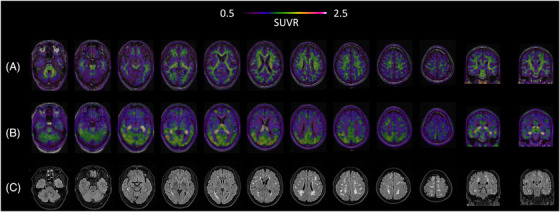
Brain imaging findings in a patient carrying p.K687Q *APP* mutation. (A) ^18^F‐florbetapir amyloid PET showing negative results. (B) ^18^F‐Florzolotau PET demonstrating asymmetric cortical uptake (SUVR maps overlaid on structural MRI). (C) T2‐FLAIR MRI. MRI, magnetic resonance imaging; PET, positron emission tomography; SUVR, standardized uptake value ratio.
